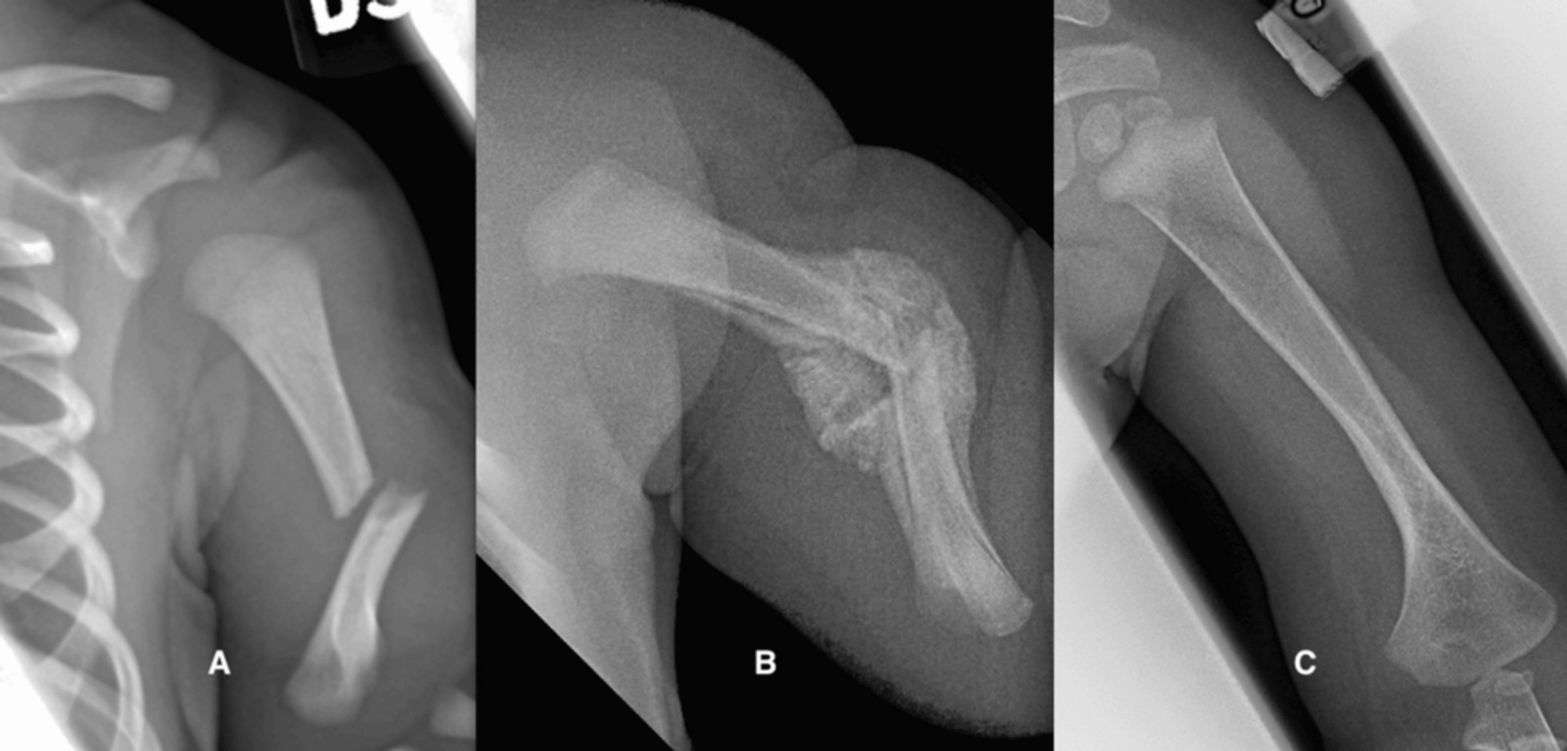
Complete remodeling of a mid-shaft humerus fracture in a neonate, sustained due to shoulder dystocia. A. Initial radiograph showing a 40-degree angulation. B. Abundant hard callus three weeks after injury. C. Normal radiograph one year later.
